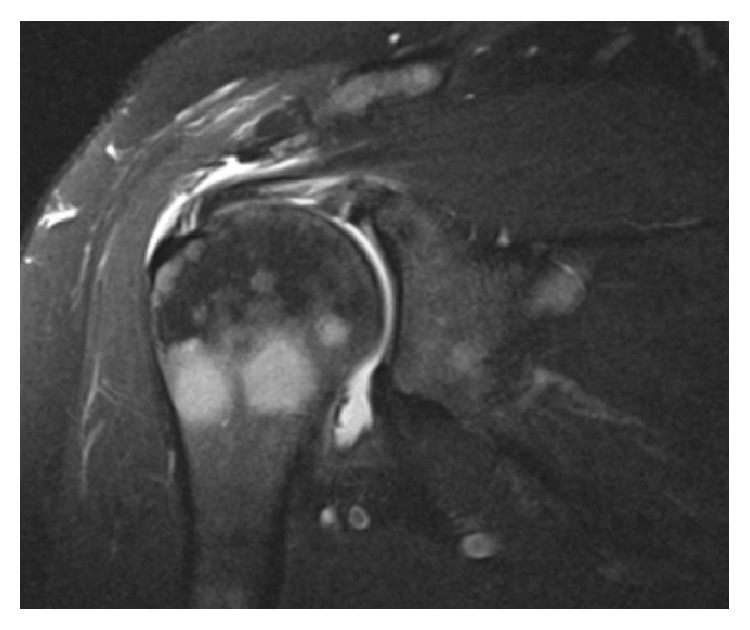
MRI demonstrating sarcoid lesions of the humerus.
